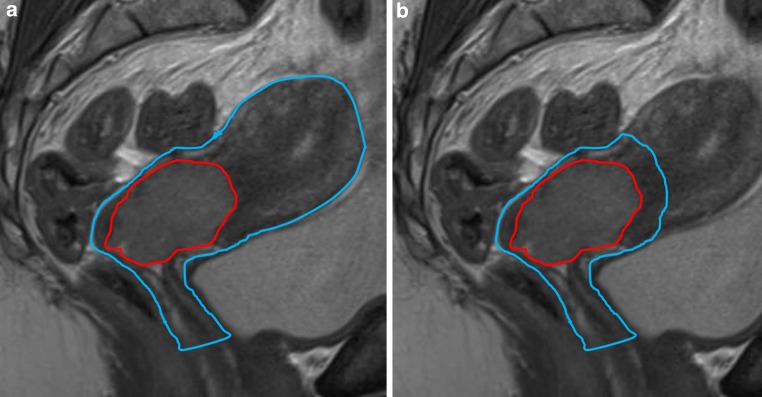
Sagittal view of T2-weighted magnetic resonance imaging with examples of the defined volumes according to the conventional definition strategy (a) and the novel definition strategy (b). In the conventional strategy (a), in addition to the GTV (*red*), the pCTV_current_ (*blue*) included the entire uterus and upper part of the vagina. According to the novel strategy, the pCTV_new_ (*blue*) excluded the uninvaded part of the uterus
